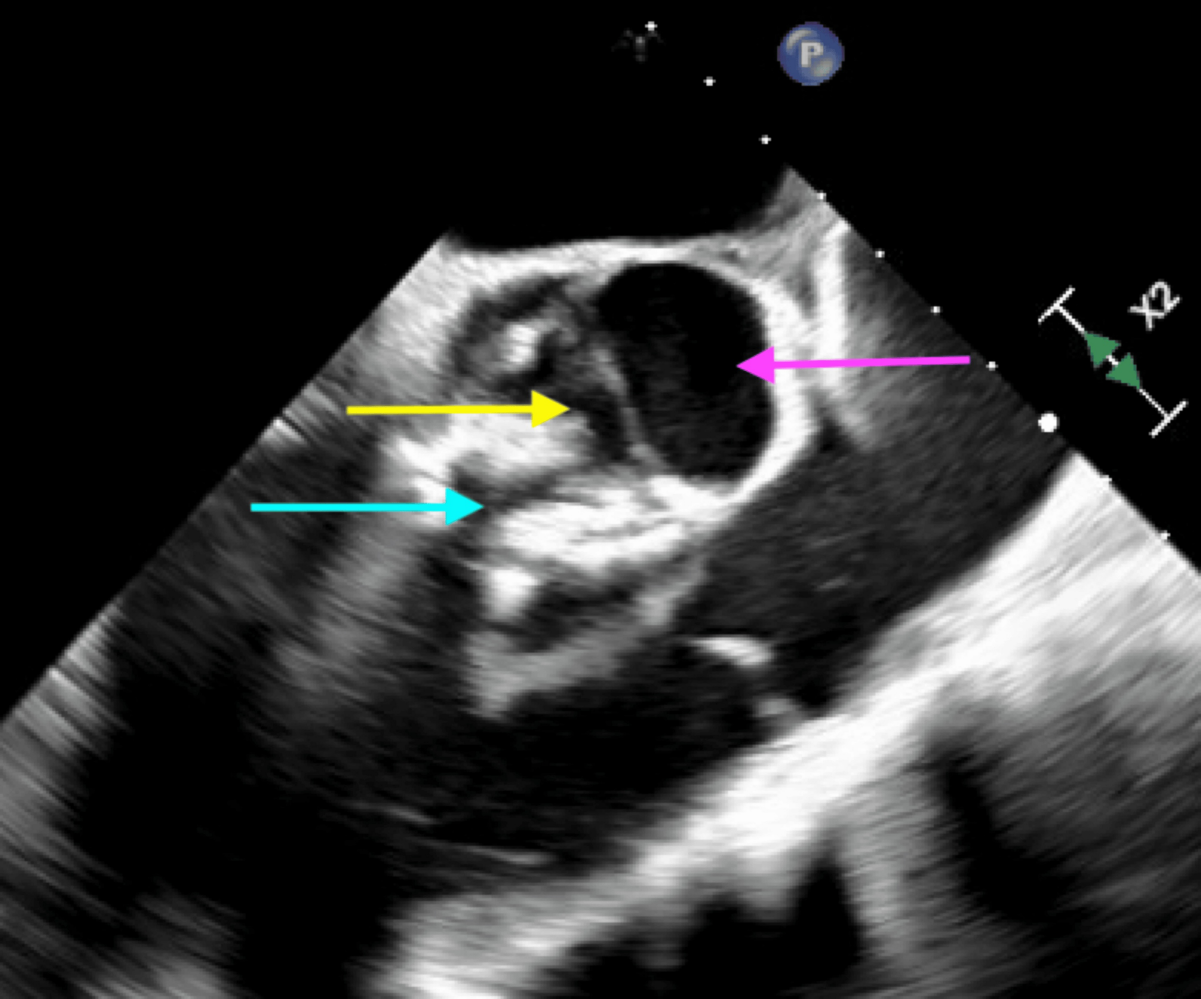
Transesophageal echocardiogram demonstrating a bicuspid aortic valve (yellow arrow). The pink and blue arrows indicate the left and right cusps, respectively.
